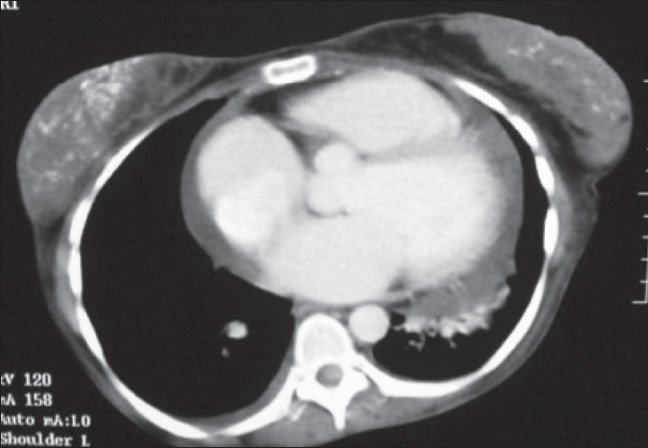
CT scan of the chest shows calcifications in both breasts and minimal pericardial effusion
